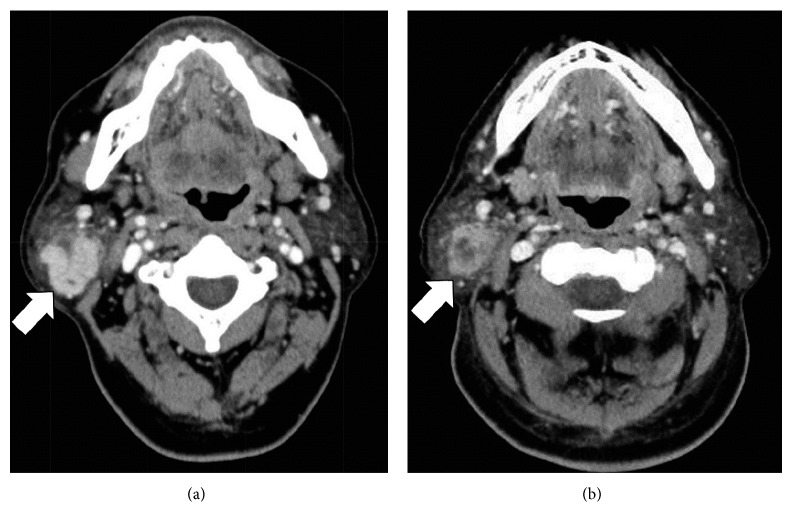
Postcontrast computed tomography (CT) findings. The right parotid gland tumor in scans obtained in our hospital (b) is smaller than that in scans obtained in our affiliated hospital (a).
